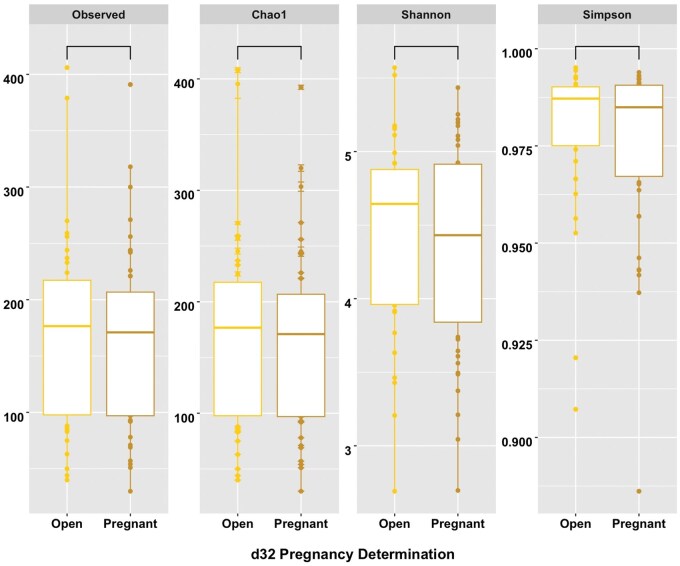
Box plots showcasing α-diversity matrices (Observed [observed OTUs], Chao1, Shannon [Shannon’s diversity index], Simpson [Simpson’s diversity index]) for differences by pregnancy status (Open and Pregnant). Alpha diversity matrices are denoted along the top, with their corresponding values on the y-axis and pregnancy status along each bottom x-axis. Pregnancy status was determined by transrectal ultrasonography on d32 post-artificial insemination.
